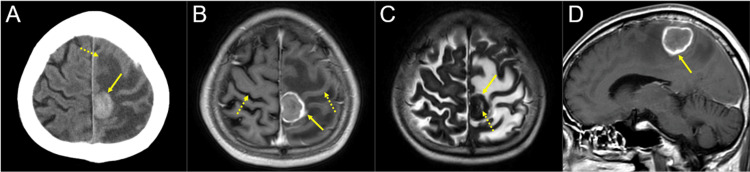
Computed tomography and magnetic resonance images at the diagnosis of the brain metastasis The images show a non-contrast-enhanced (CE) computed tomography (CT) image (A); CE-T1-weighted images (WIs) (B, D); a T2-WI (C); axial images (A-C); and a sagittal image (D). (A-D) These images are shown at the same magnification and coordinates under co-registration and fusions. (A) A high-density, well-demarcated solid mass lesion (arrow in A) located in the left parasagittal para-central sulcus region and associated with extensive perilesional edema (dashed arrow in A) in the ipsilateral fronto-parietal lobes (B, D). The mass itself is slightly enhanced, whereas the surrounding region is markedly enhanced with slight exudation of contrast media into the surrounding parenchyma (arrows in B, D). The bilateral central sulci are indicated by dashed arrows in B. (C) On T2-WIs, the mass (arrow in C) is generally low-intensity, containing the high-intensity region (dashed arrow in C), suggestive of central necrosis.
